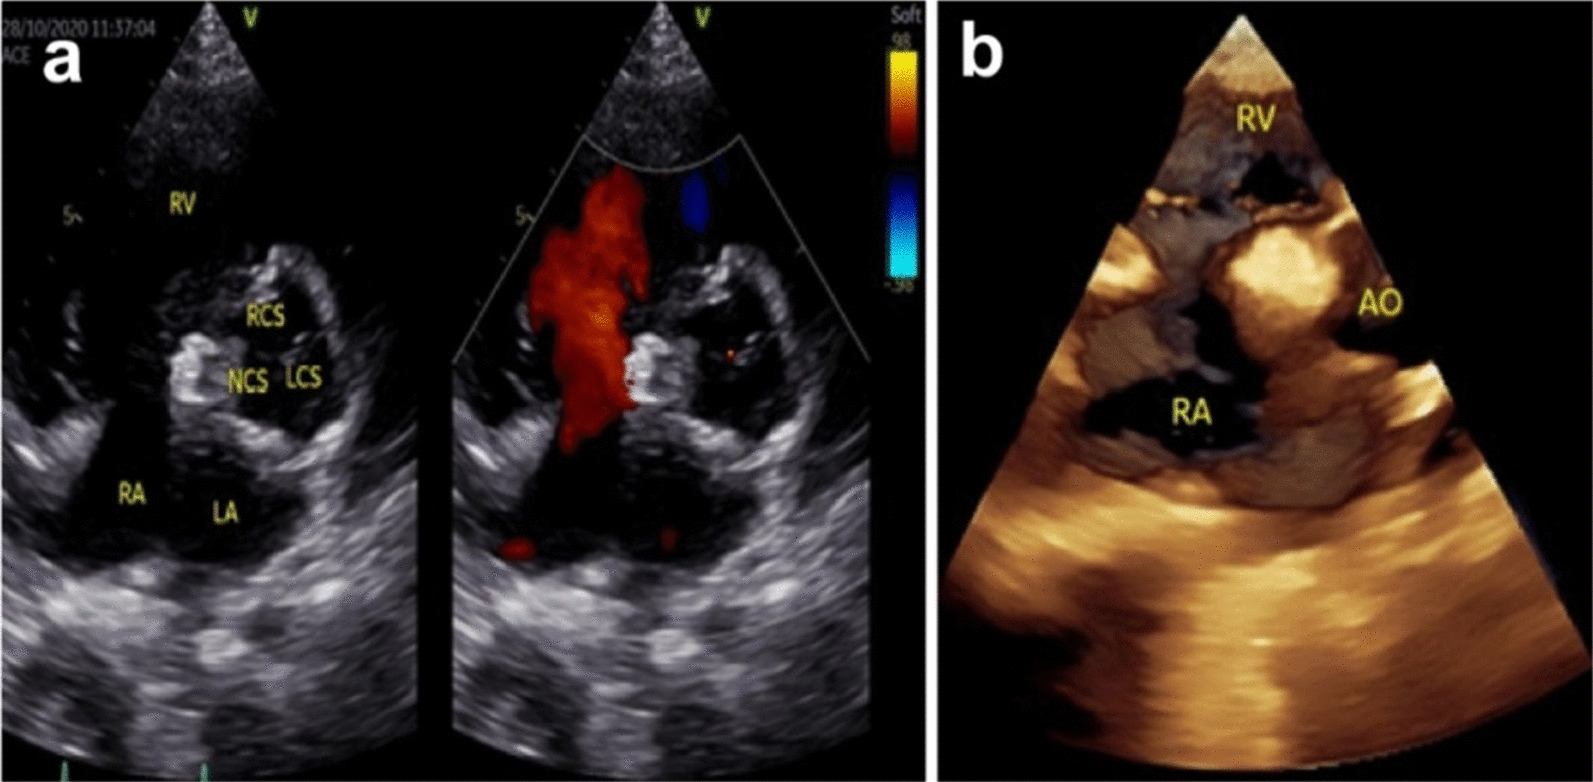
a Echocardiography with colour Doppler showing the closure device, and there was no shunt from the aorta to the cardiac chamber; b Echocardiography with a 3D imaging view of the aortic root showed the closure device. LCS, left coronary sinus; NCS, noncoronary sinus; RCS, right coronary sinus; RA: right atrium; RV, right ventricle; LA: left atrium
